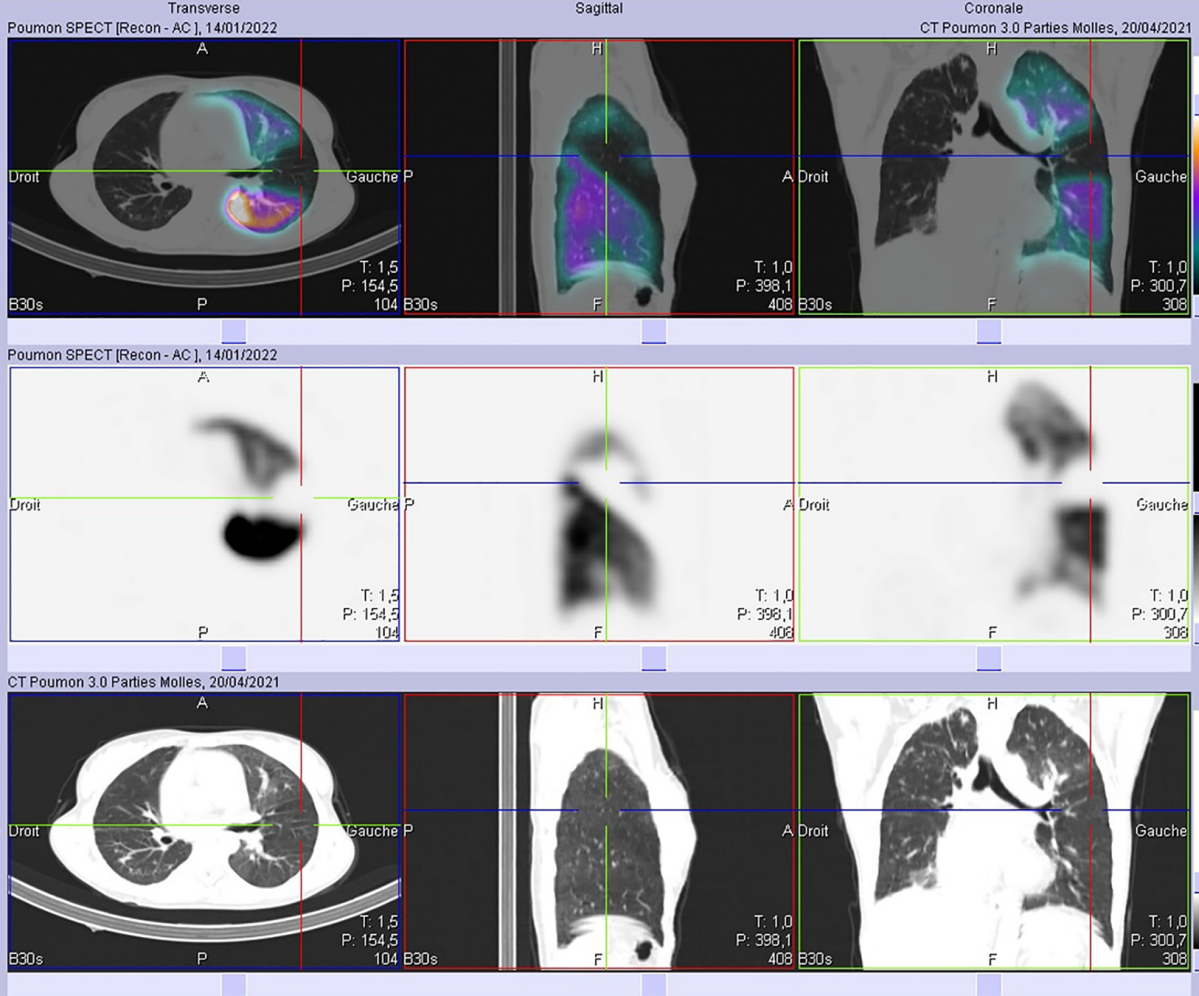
No abnormal parenchymal findings were seen in both lung fields adjacent to the defects on SPECT-CT images.
